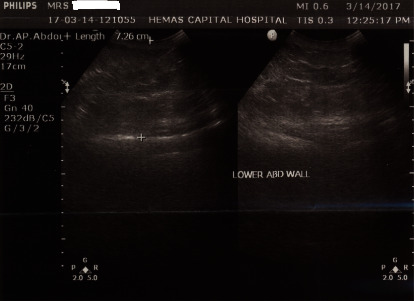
Ultrasound scan of the abdomen showing extensive abdominal wall oedema.
